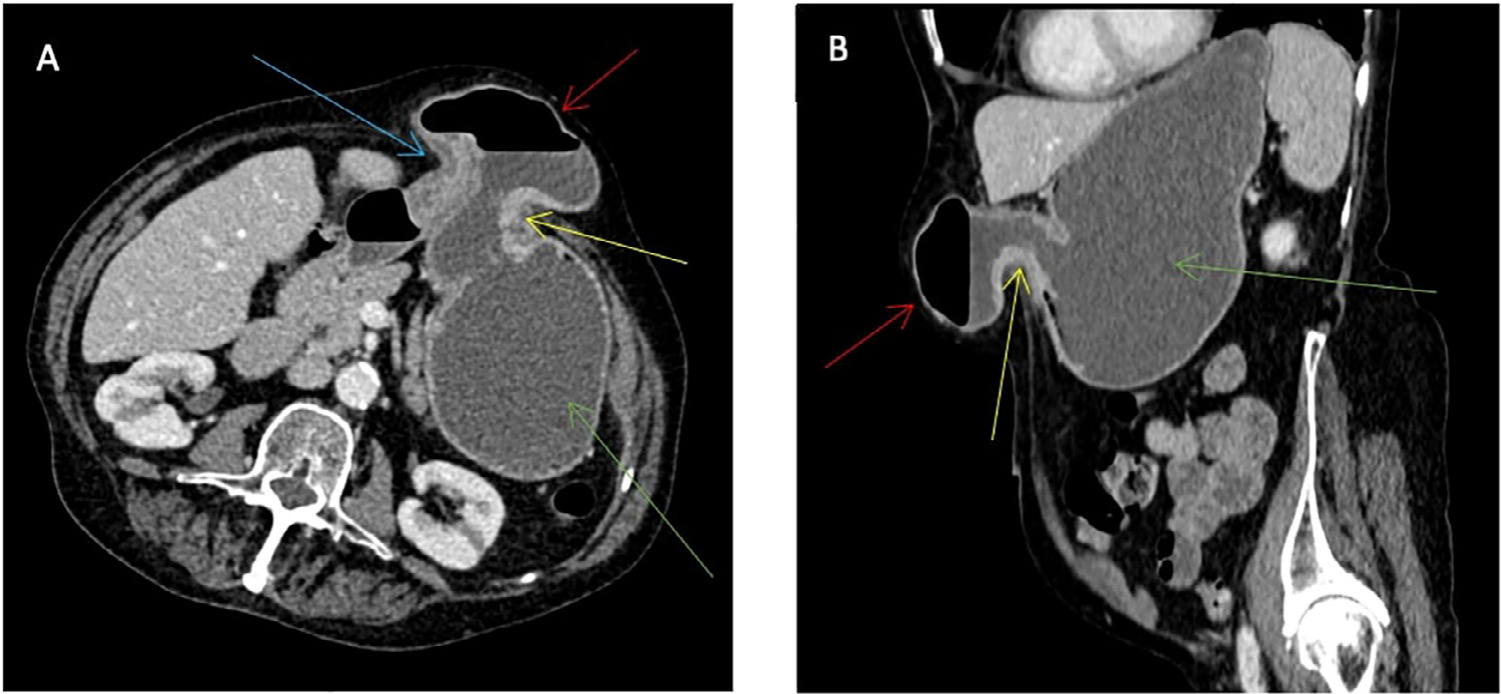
CT scan of the abdomen and pelvis with intravenous contrast in the portal venous phase. Axial (A) and coronal reformatted (B) images. These demonstrate herniation of the distal stomach through the anterior abdominal wall. The neck of the hernia is narrow and contains the distal body entering the hernial sac (yellow arrow) and the pylorus of the stomach exiting the hernial sac and re-entering the abdomen (blue arrow). There is narrowing of the lumen at both these points. The proximal stomach (green arrow) is distended, and fluid filled. The red arrow shows the hernial sac.
